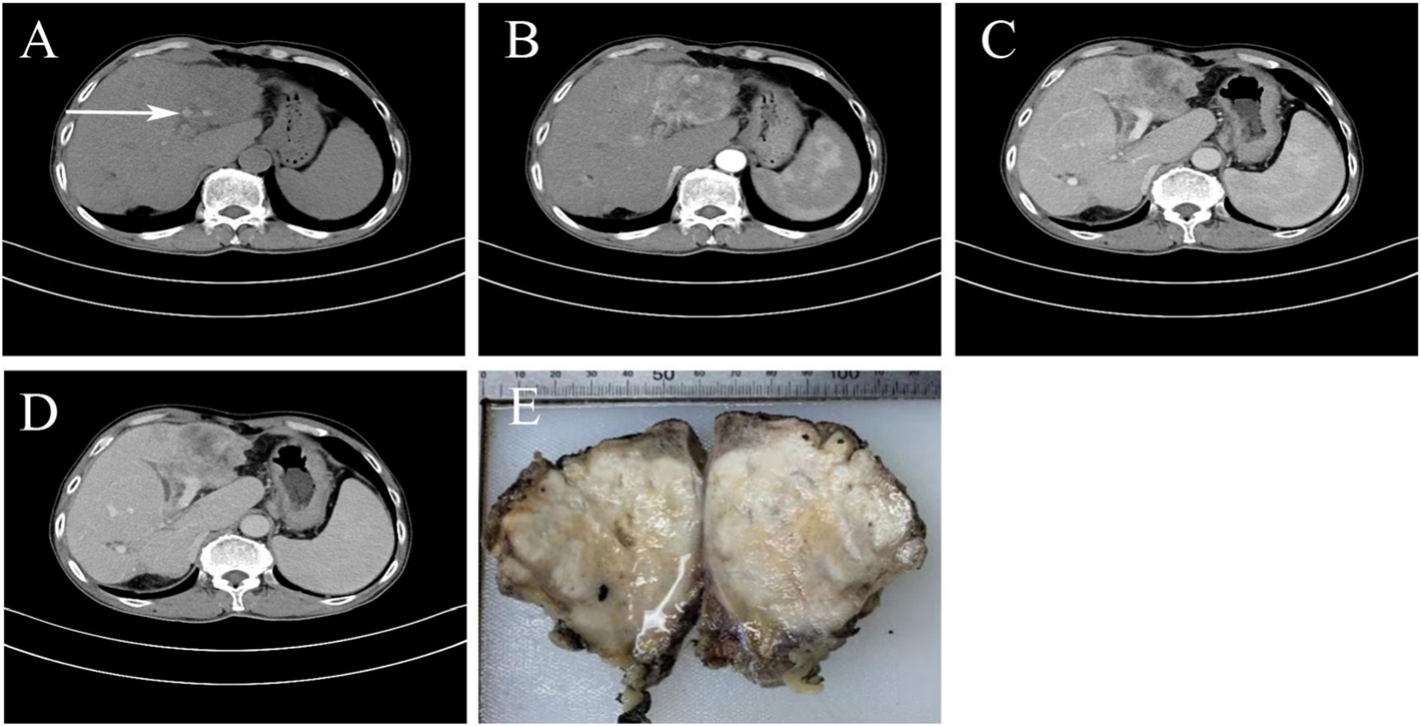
Results of preoperative CT, postoperative gross examination. a Abdominal non-contrast CT scan revealed a low density mass measuring approximate 10 cm in diameter at the left hepatic lobe and intrahepatic bile ducts with multiple stones (white arrow). b Enhanced CT scan (arterial phase) revealed a heterogeneous enhanced mass in the left lobe of the liver. c Enhanced CT scan (portal phase) revealed a persistent inhomogeneous enhanced mass and formation of tumor thrombus in the left branch of portal vein. d Enhanced CT scan (delayed phase) revealed a persistent inhomogeneous enhanced mass. e Gross examination of the resected liver mass indicated an irregular, white solid tumor without a fibrous capsule. The border between the tumor and normal liver tissue was indistinct
